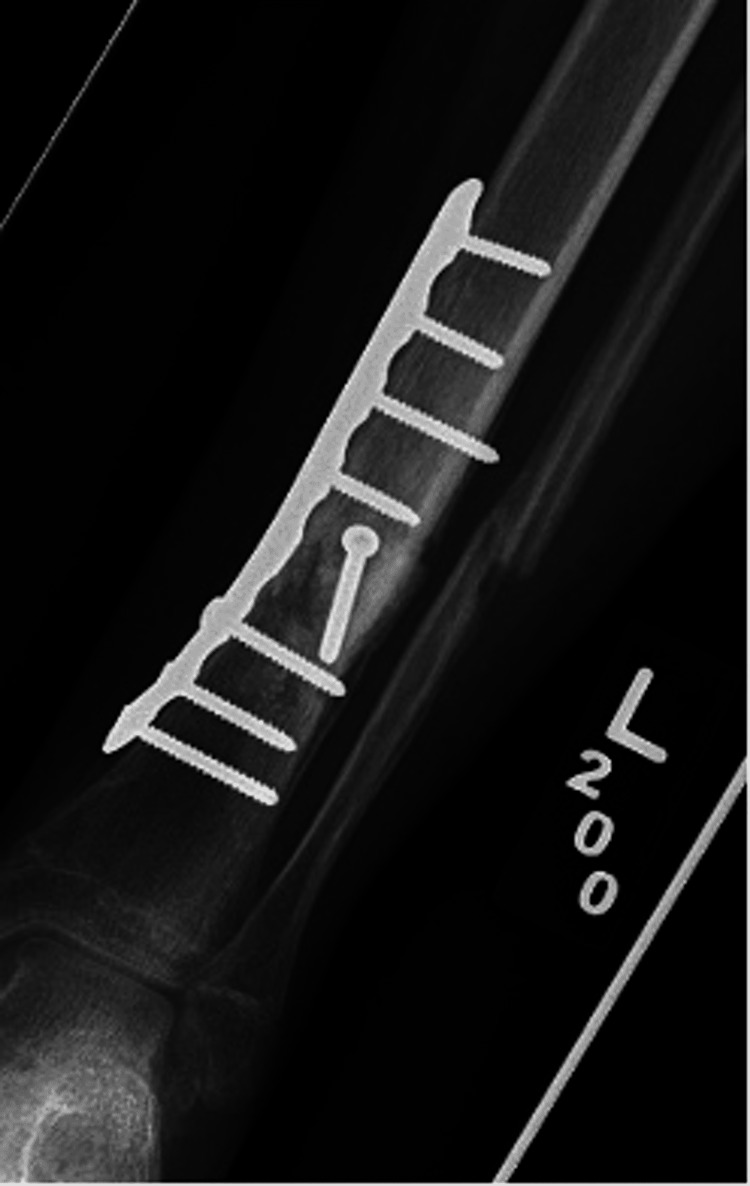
Post-operative anterior-posterior tibia-fibula radiographs of revision open reduction and internal fixation with removal of flexible nails of tibial shaft.
